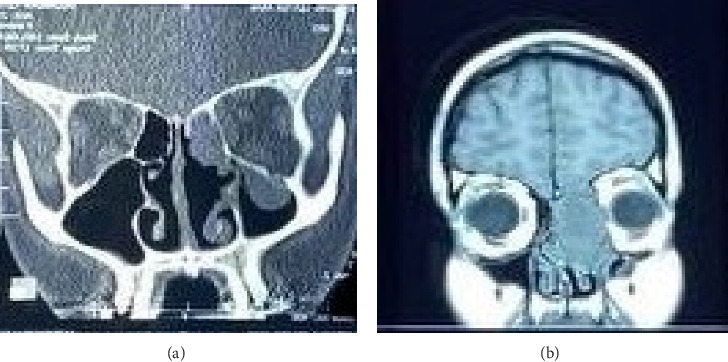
The CT (a) and T1-weighted MRI (b) of the patient before surgery.
